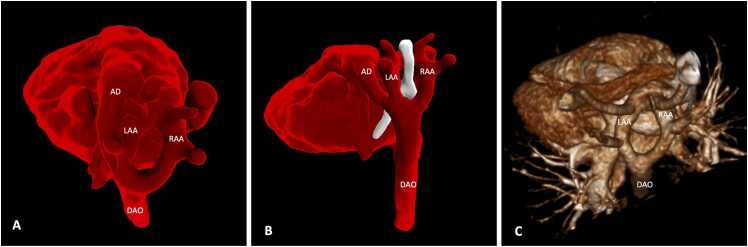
DAA with balanced arches. Segmentation of motion-corrected black-blood fetal CMR data (A, B) at 30 weeks gestation shows a complete DAA with similar size left and right aortic arch encircling trachea (white mesh) and esophagus. The LAA courses posteriorly to join the descending aorta. The left-sided arterial duct can also be appreciated inferior to the LAA. Postnatal contrast-enhanced CT (C) at 3 months shows balanced aortic arches. The left arterial duct is no longer patent and therefore not visible on contrast-enhanced CT. *AD* arterial duct, *DAO* descending aorta, *RAA* right aortic arch, *LAA* left aortic arch, *DAA* double aortic arch, *CMR* cardiovascular magnetic resonance, *CT* computed tomography.
